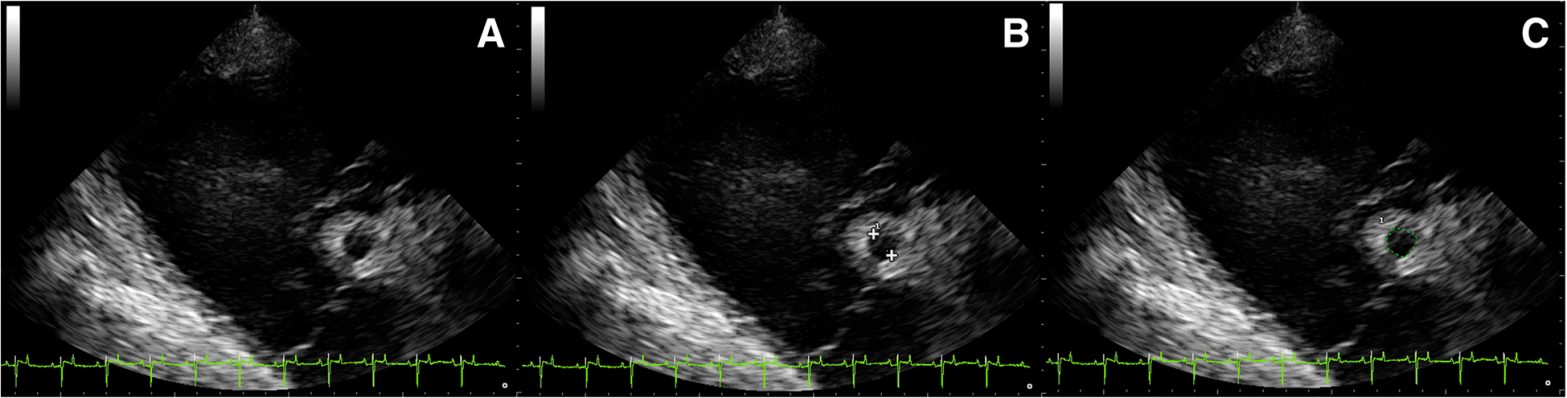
(a) 2D ultrasound image portraying the RCA (right parasternal long axis view). (b) Imaging of the inner lumen RCA diameter using the inner to inner method. (c) Measuring the inner lumen area of the RCA using an ultrasound contour caliper
